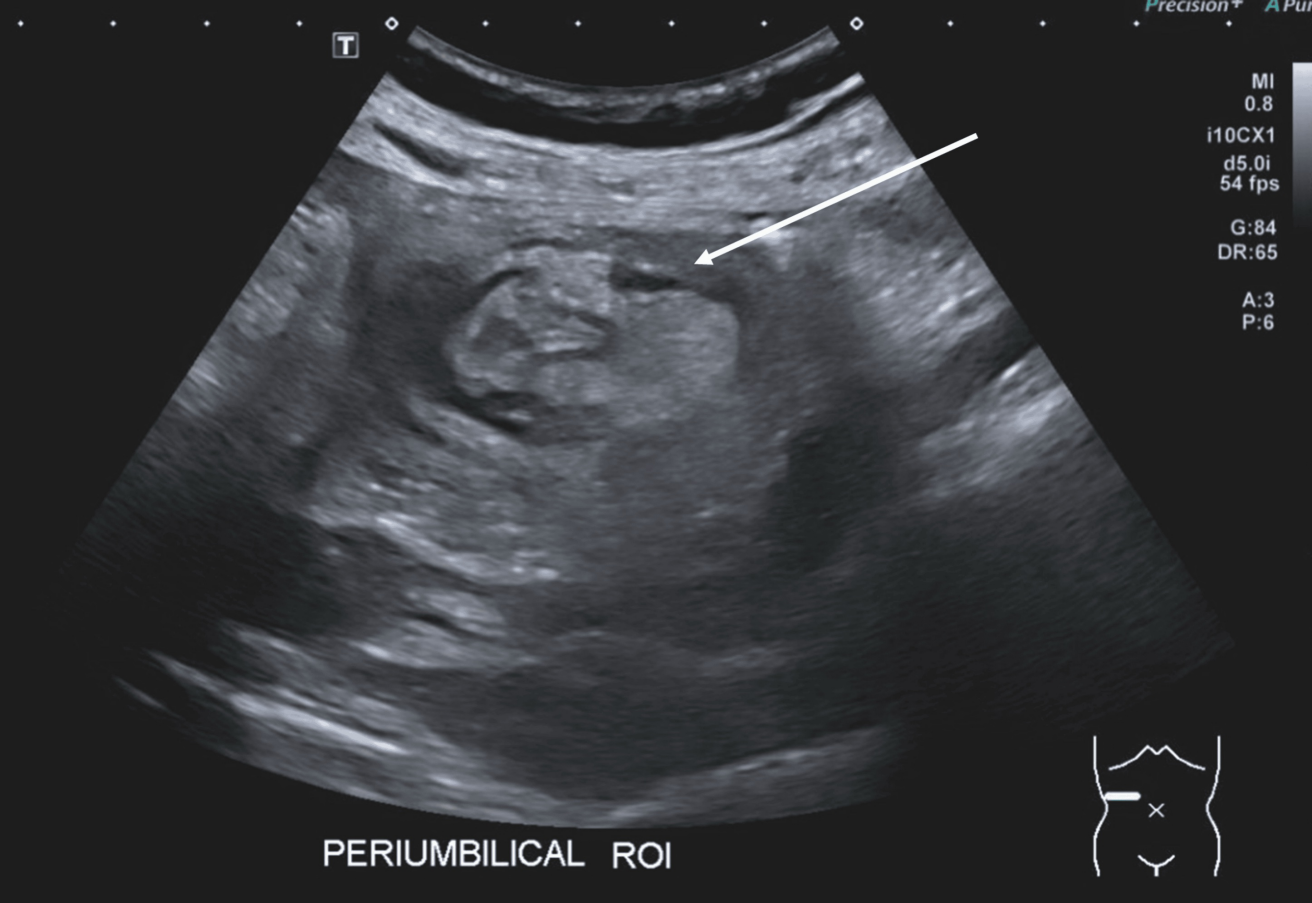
Ultrasound showing the characteristic donut sign seen in intussusception This is characterized by concentric alternating echogenic (bright) and hypoechoic (dark) bands; these bands are formed by the layers of the intestine involved in the intussusception.
